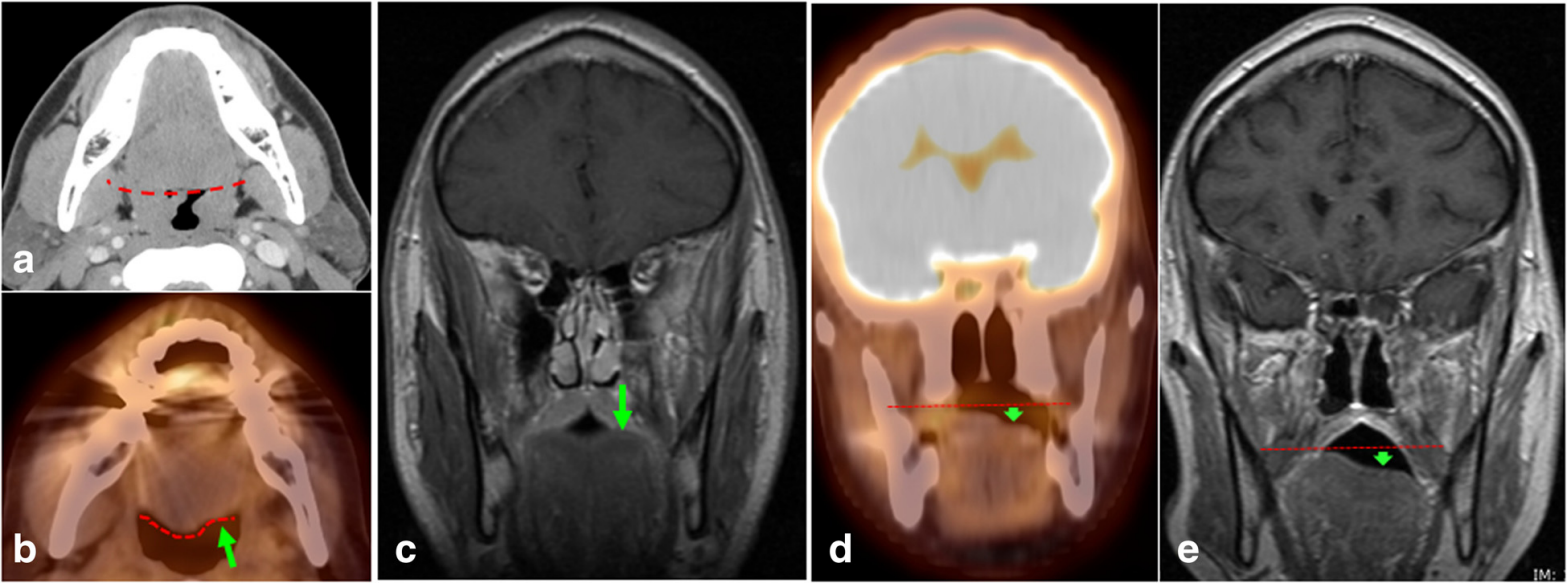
A 41 year-old-male patient diagnosed with left sided nasopharyngeal carcinoma and intracranial involvement. Axial CT of the neck (a) at the time of diagnosis (5/2005) showing normal posterior contour of the tongue (red line). The patient received radiotherapy and chemotherapy ending in 2006. Follow-up axial fused PET/CT images of the neck region (b) on (8/2013) shows interval atrophy of the left hemi-tongue, with abnormal posterior contour (green line). Post contrast coronal T1 WI before treatment (c), coronal fused PET/CT and coronal contrast enhanced MRI T1W images after treatment (d, e) confirming the left hemi tongue atrophy (arrow). Clinically, the patients tongue deviates to the left. The constellation of findings is consistent with post radiation hypoglossal neuropathy
